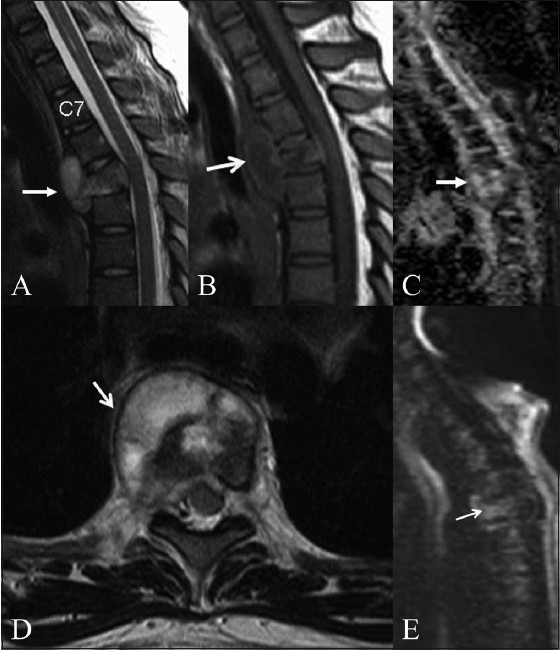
Tuberculosis of the spine. T2W (A), T1W (B) axial MRI images in a 12-year-old girl with upper dorsal spine tuberculosis show a small prevertebral abscess (arrow). Wedging of the D2 vertebral body is seen with marrow involvement. Sagittal ADC map (C) sagittal and T2W (D) and diffusion (E) images show increased diffusion (arrow) in the involved vertebra (ADC: 1.35 × 10^−3^ mm^2^/s).
